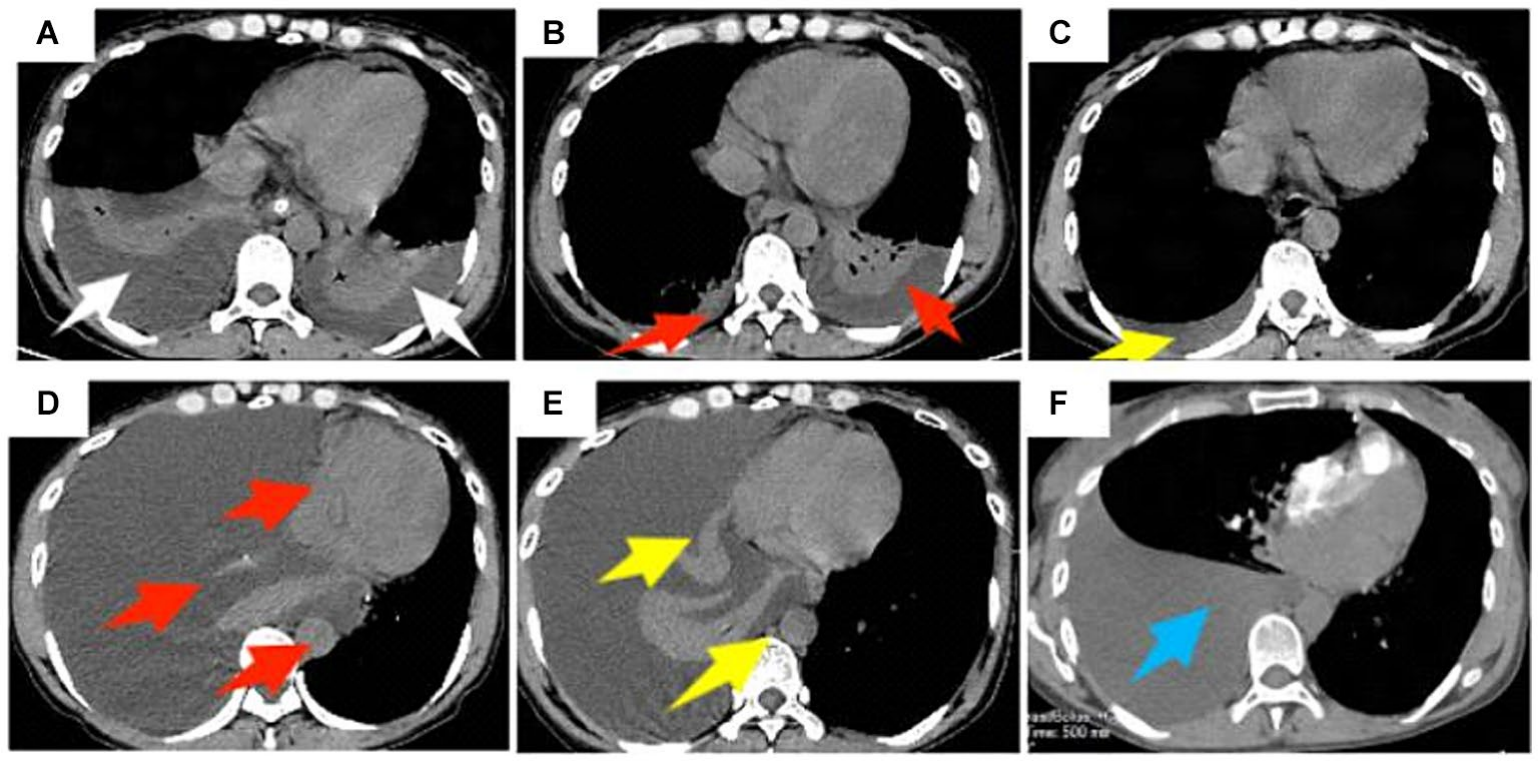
A 47-year-old female patient underwent obot-assisted laparoscopic splenectomy combined with pericardial devascularization (LSPD). (A) Chest CT 4 days after LSPD showed bilateral pleural effusions and most of the lower lobes of both lungs were atelectatic (white arrows); (B) percutaneous percutaneous drainage of the right pleural effusion was performed, and a follow-up CT 8 days after the operation showed a decrease in the bilateral pleural effusions (red arrows); (C) Chest CT 47 days after the operation showed a small amount of effusion in the right pleural cavity (yellow arrow); (D) The mediastinal window of the chest CT on day 116 postoperatively showed a large right pleural effusion, complete atelectasis of the right lung, and left shift of the trachea, heart, and mediastinum (red arrow); (E) the patient underwent percutaneous right pleural effusion puncture and drainage, and chest CT on day 129 postoperatively still showed a large right pleural effusion (yellow arrow); (F) chest CT on day 137 postoperatively showed a large right pleural effusion but less than before (blue arrow).
